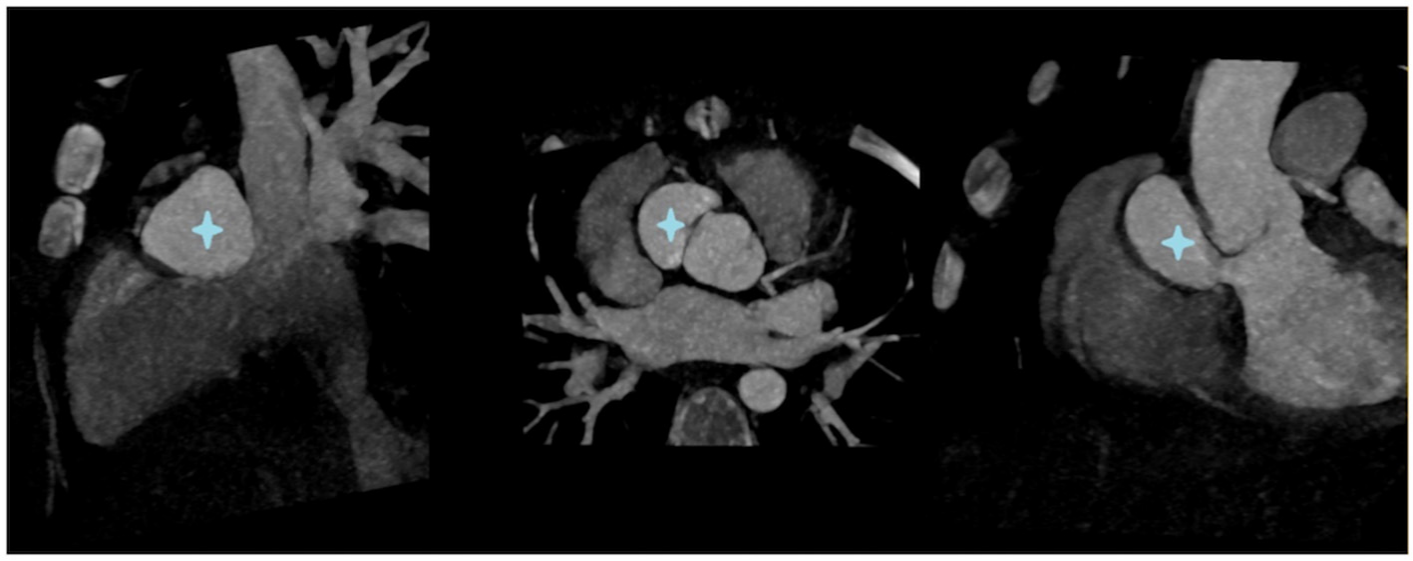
CT scan showing a para-aortic pseudoaneurysm (
